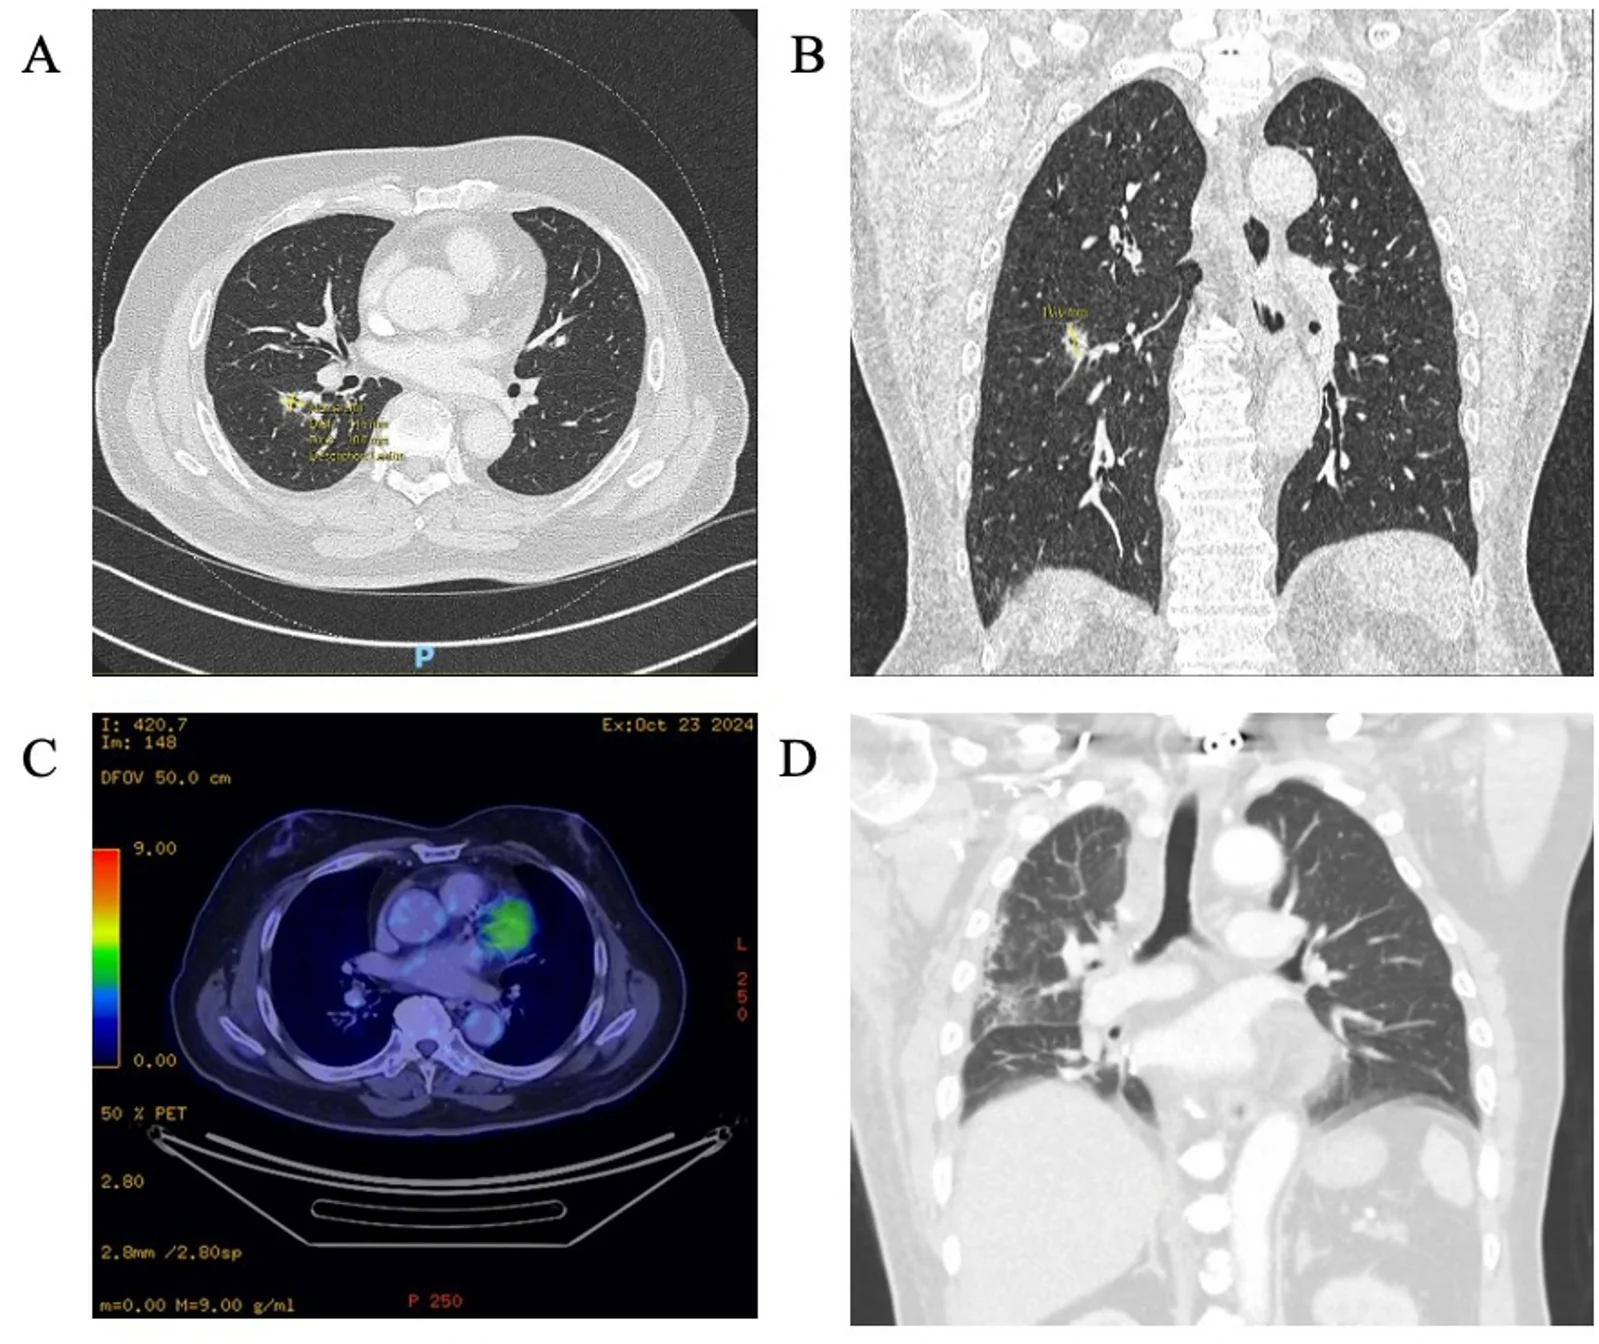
(A-B) CT thorax showed a spiculated sub-solid peri-bronchial nodule in the superior segment of the right lower lobe. (C) PET/CT scan showed a 2.2 cm right lower lobe nodule with mild FDG-avidity and no metastasis (D) CT thorax showed no definite rim-enhancing collection detected at the right chest wall with a new small right pleural effusion
